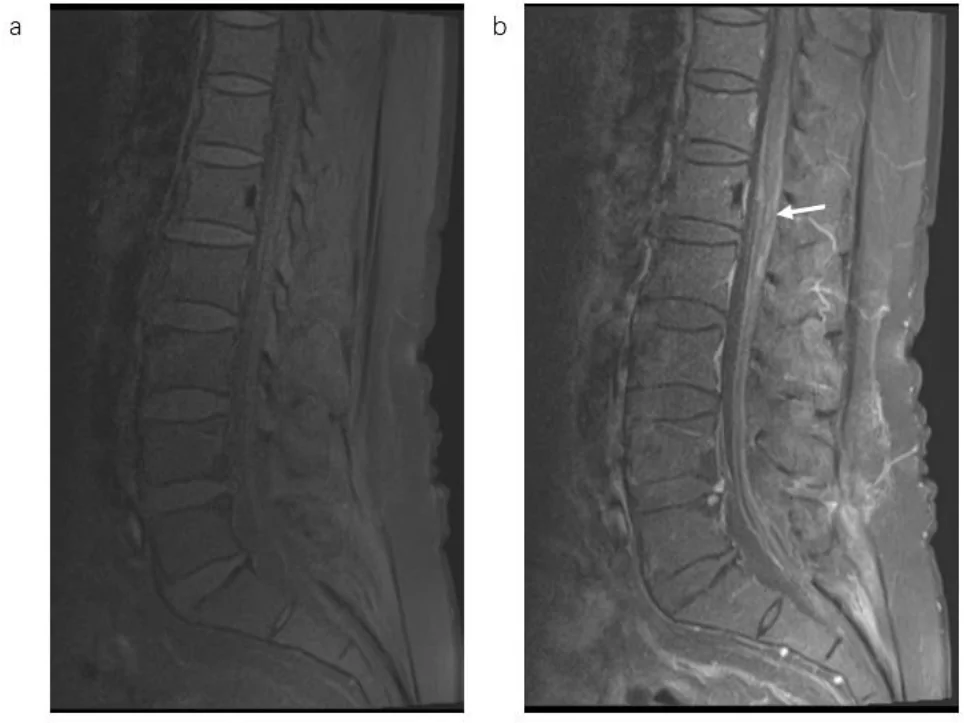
(a, b) T1-weighted and contrast-enhanced T1-weighted MR images of the patient's spinal cord, respectively, demonstrating mild linear and patchy enhancement involving the conus medullaris.
